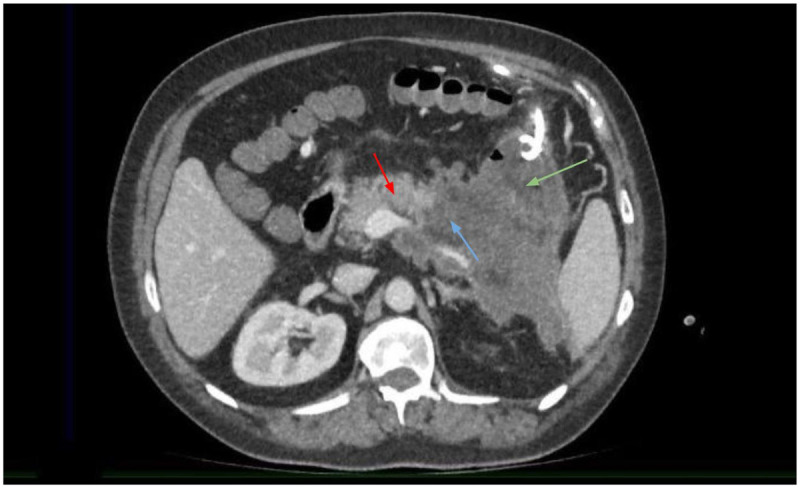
Contrast-enhanced CT of the abdomen: red arrow—viable pancreatic parenchyma; blue arrow—extensive necrosis of the pancreatic body and tail; green arrow—fluid collections within the omental Bursa.
